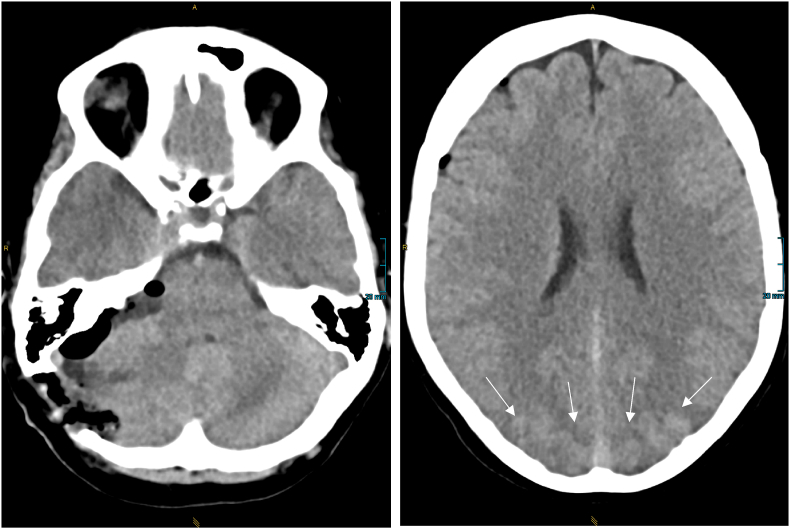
Non enhanced cranial computed tomography post resection showing subtle hypodensity of both occipital poles (marked with asterisks) as a first sign of PRES.
